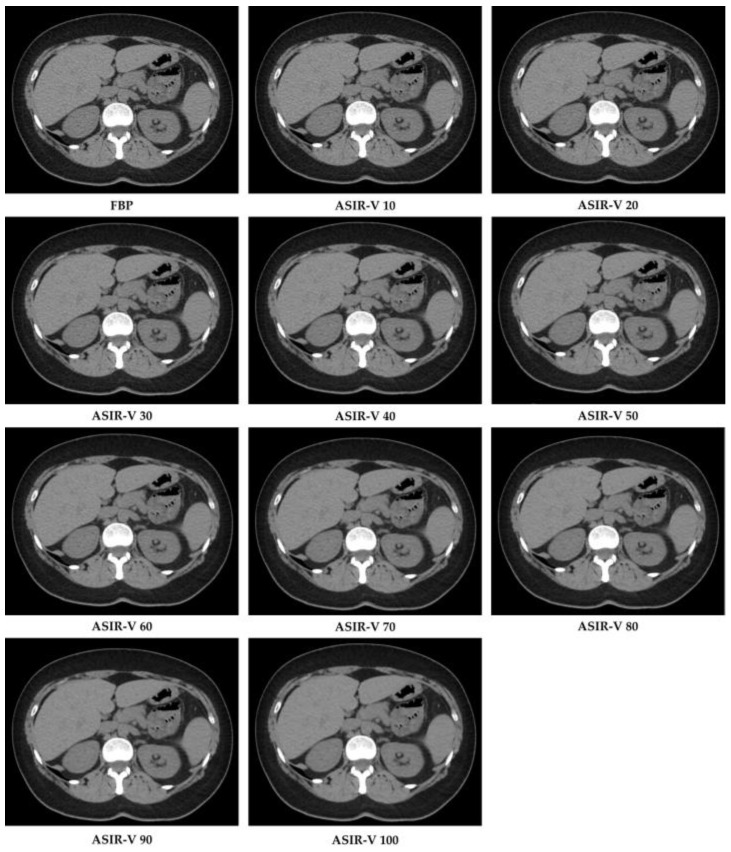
CT image reconstructions at filtered back projection (FPB) and at different Adaptive Statistical Iterative Reconstruction-V (ASIR-V) levels.
